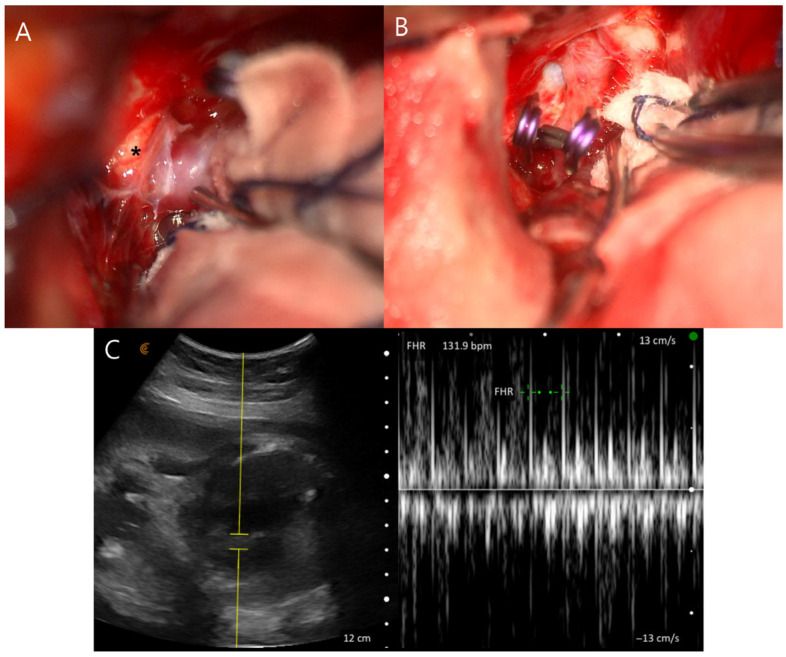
Intraoperative findings. (A) Following left frontotemporal craniotomy and Sylvian dissection, the aneurysm at the left posterior communicating artery was confirmed, with compression of the third cranial nerve (asterisk). (B) The aneurysm was completely clipped. (C) Postoperative ultrasonography confirmed fetal stability. The yellow area represents the sample region used for Doppler flow analysis.
